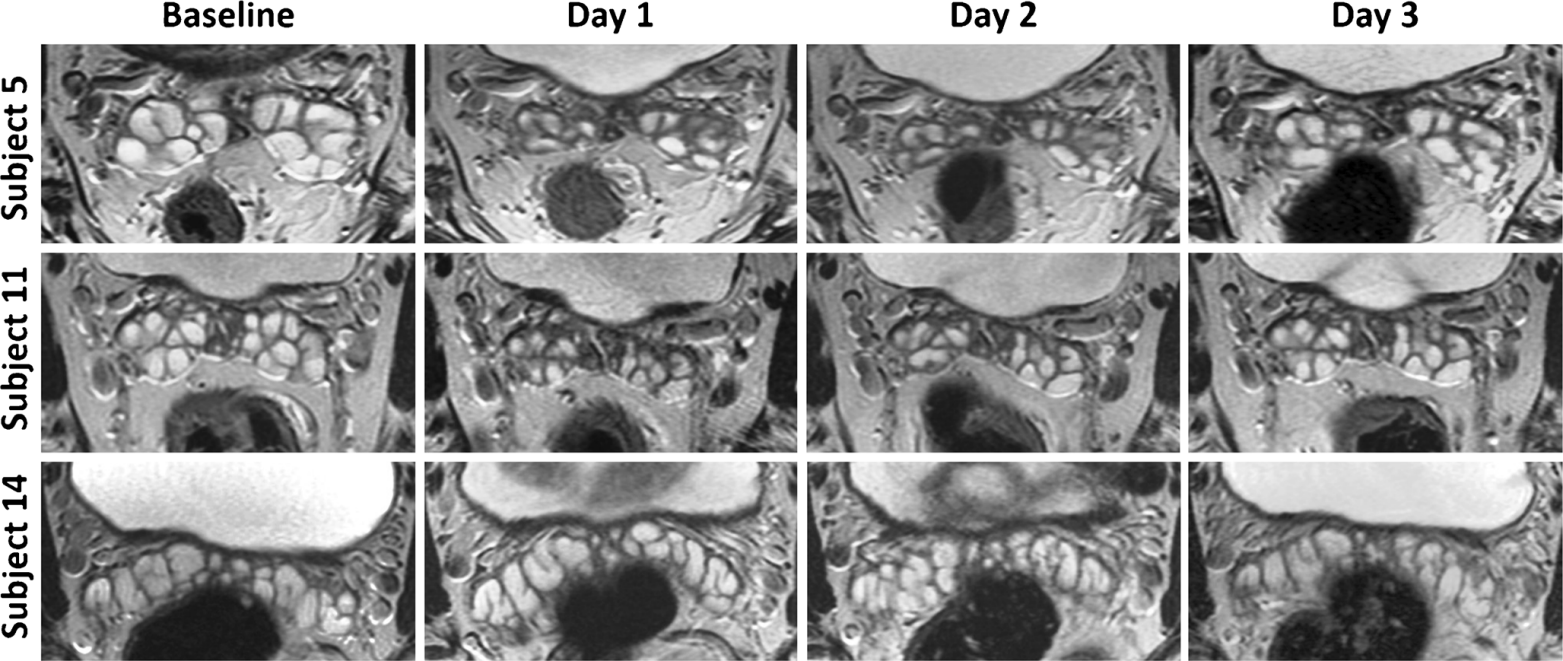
Examples of seminal vesicle volume change over time. Axial T2-weighted MR imaging performed at baseline (minimum 3 days post-ejaculation), and post ejaculation on days 1, 2 and 3. *Top row* subject 5 (aged 27) shows significant reduction in volume from a baseline of 9.7 cm^3^ to 3.6 cm^3^ on day 1 and subsequently to 4.7 cm^3^ and 6.9 cm^3^ on days 2 and 3, respectively. *Middle row* subject 11 (age 53) shows similar reductions from 7.0 cm^3^ at baseline to 3.3 cm^3^, 4.2 cm^3^ and 5.3 cm^3^ on days 2, 3 and 4, respectively. Note apparent increase in wall thickening on day 2, due to relative underdistension. *Bottom row* subject 14 (age 36) shows little difference in SV volume over the four scans: 10.6 cm^3^, 10.0 cm^3^, 10.5 cm^3^ and 10.9 cm^3^, respectively
